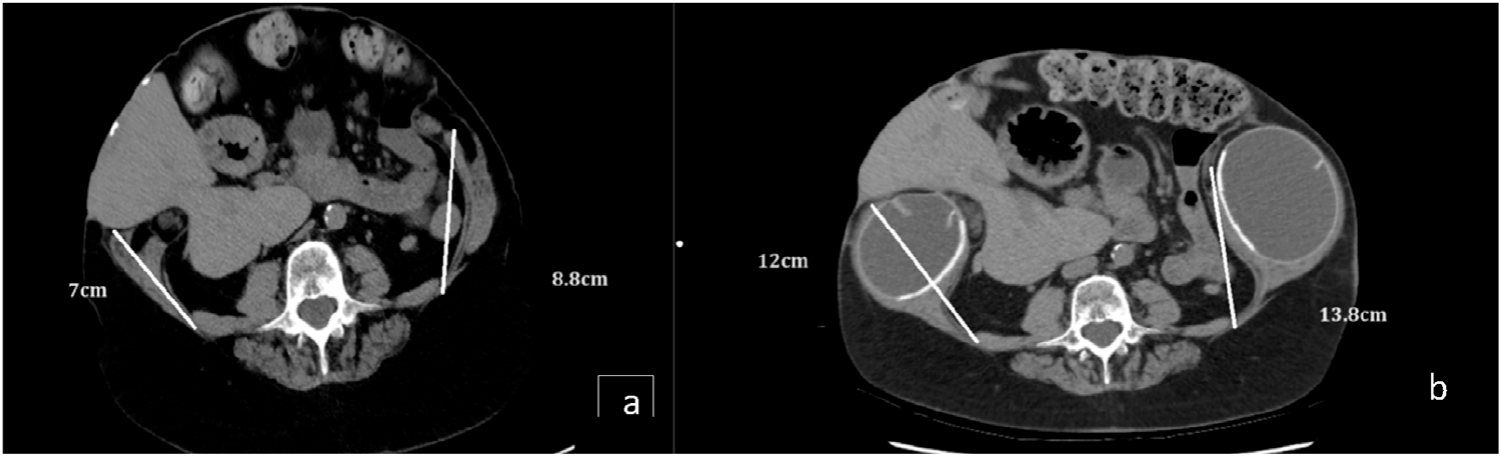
(A) Axial CT before expansion axial CT. (B) Axial CT demonstrating the additional length gained in the oblique muscles and aponeurosis following full expansion and botox.
